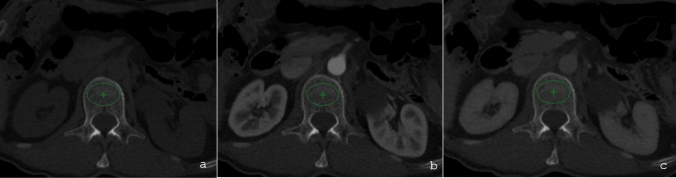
Example of three axial CT images at the level of the first lumbar vertebra acquired in the pre-contrast (a), arterial (b) and portal venous (c) phases. A region of interest containing trabecular bone (excluding cortical bone) was traced
